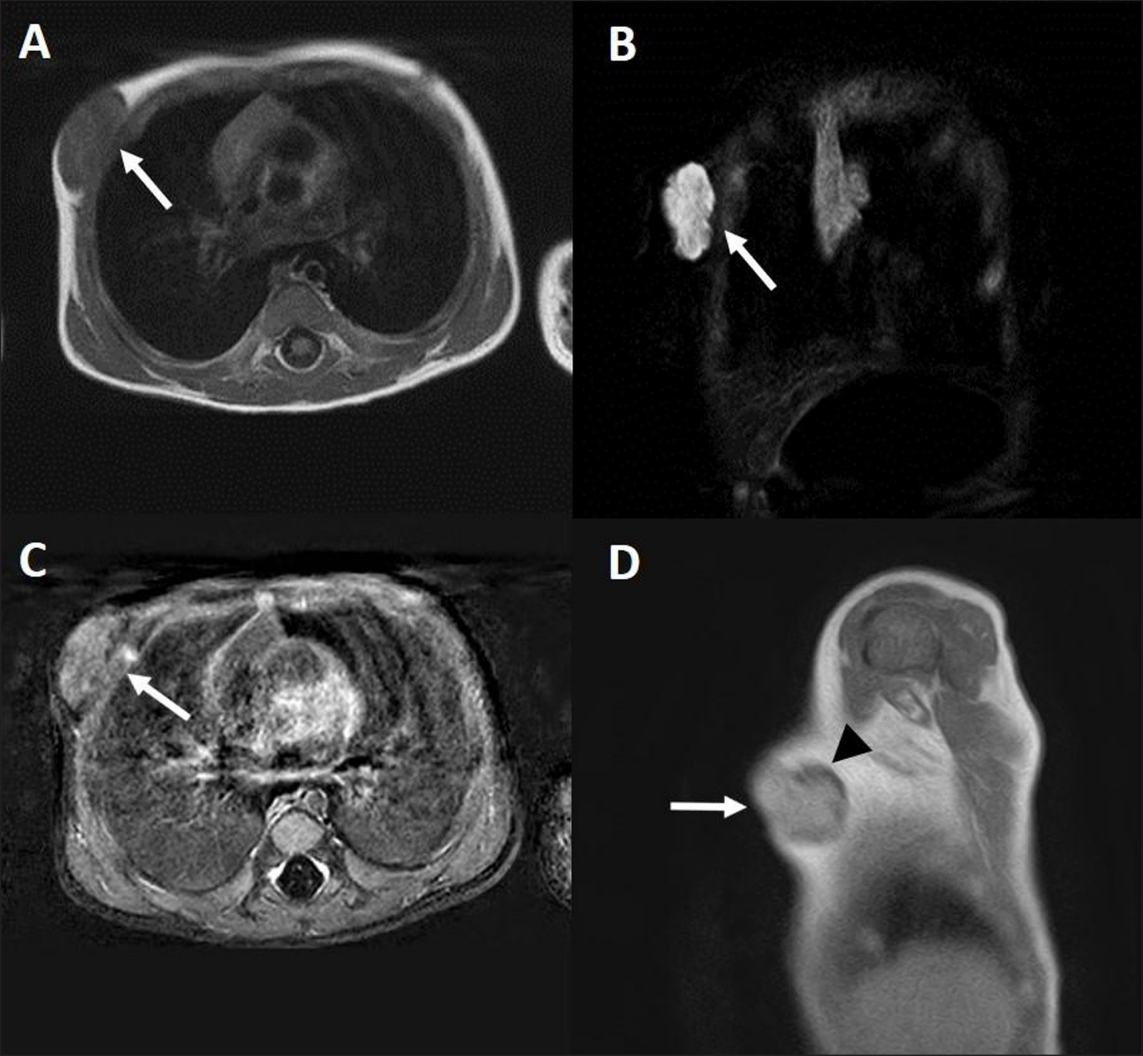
MRI examination (A-D) with axial T1 (A), coronal STIR (B), axial T1-substraction (C) and sagittal T1 post-contrast (D) images. The mass is highlighted with arrows. Note the non-enhancing peripheral cysts (arrowhead) on image D.
